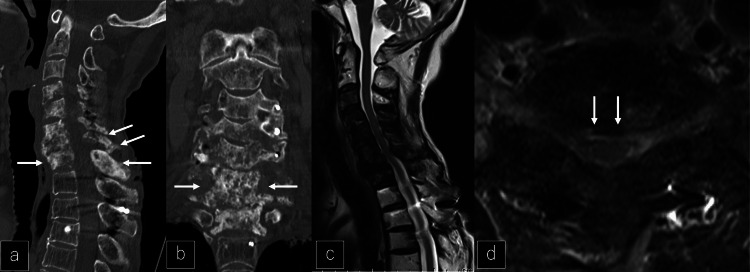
CT and MRI after the resumption of denosumab a, b: Sagittal and coronal CT after the resumption of denosumab showing bone recalcification from C4-T1 (white arrows). c, d: Sagittal and axial MRI after the resumption of denosumab showing the release of spinal cord compression (white arrows).
